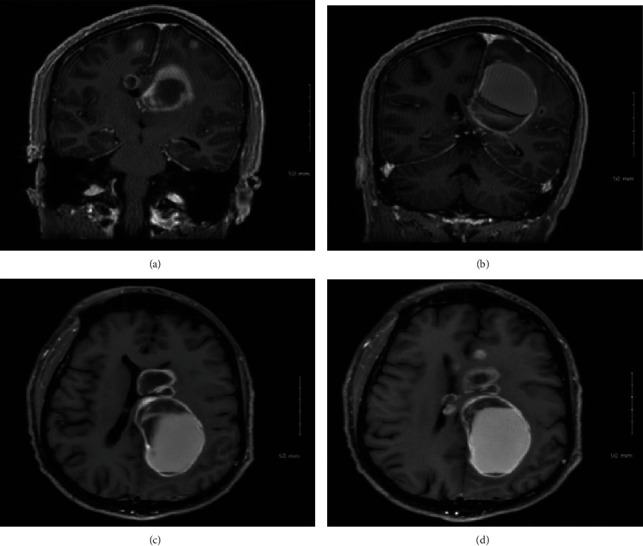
MRI images of the patient's brain. (a, b) T1 postcontrast coronal sequences of the brain showing multiple cystic lesions of varying sizes in the cerebral hemispheres. (c, d) T1 postcontrast axial sequences of the brain showing multiple cystic lesions of varying sizes in the cerebral hemispheres.
